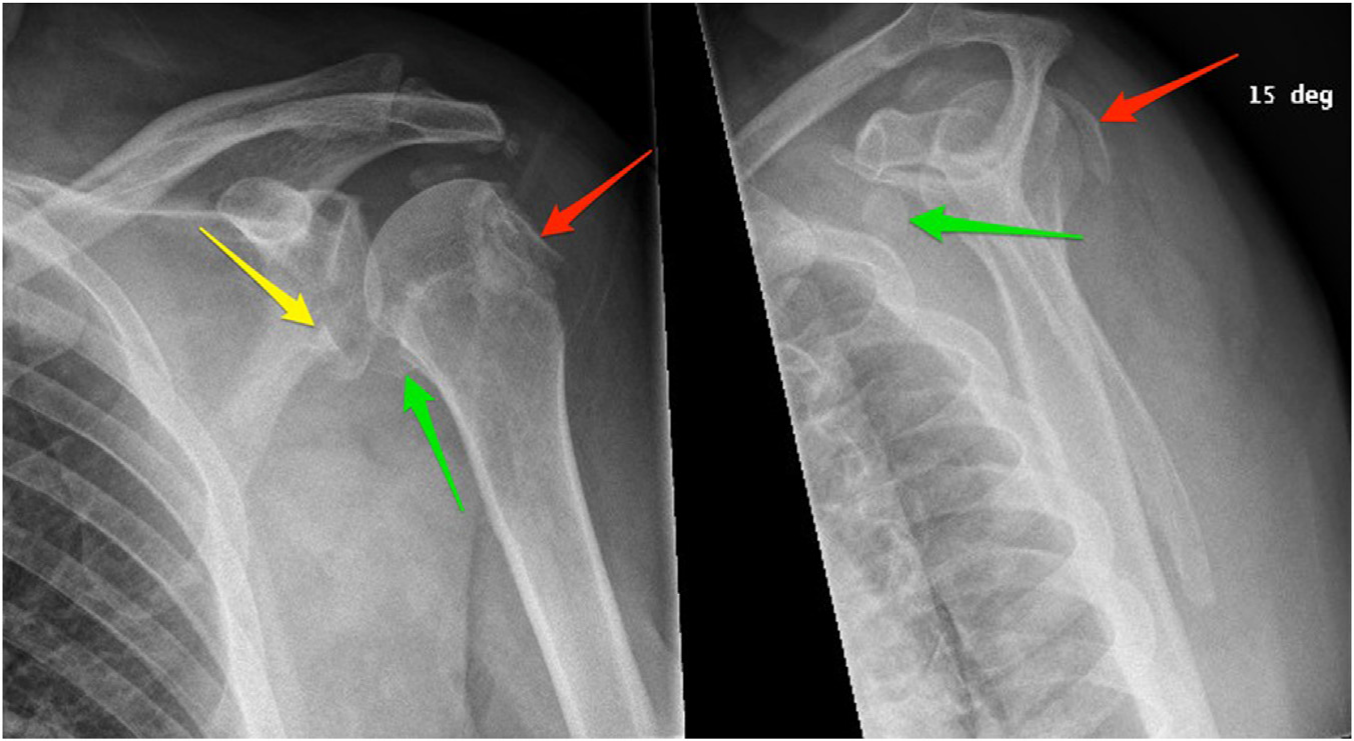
Radiography (2 views) from orthopedic surgery consultation. *Red arrows* indicate Hill–Sachs deformity, *yellow arrow* indicates bony Bankart lesion, and *green arrows* indicate coracoid avulsion.
